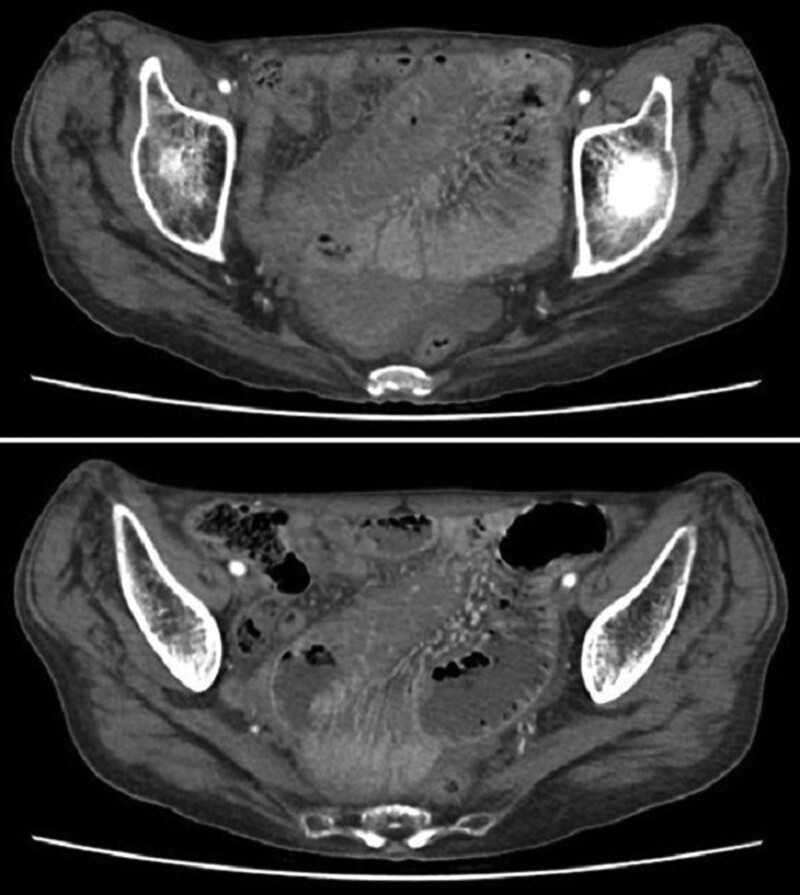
Computed tomography disclosed disproportional dilatation of the segmental small bowel, poorly enhanced mucosal circulation with severely swollen mucosa and near-total obstruction of the intestinal lumen at the level of the jejunum.
